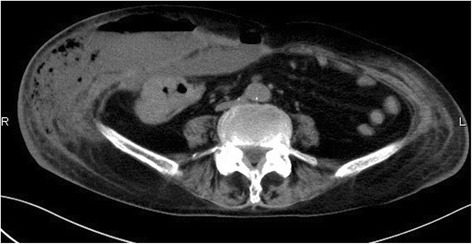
CT demonstrating a large abdominal wall abscess containing air. There was no apparent connection between the abdominal wall abscess and the abdominal cavity, although the wall of the ascending colon adjacent to the abscess was thickened
